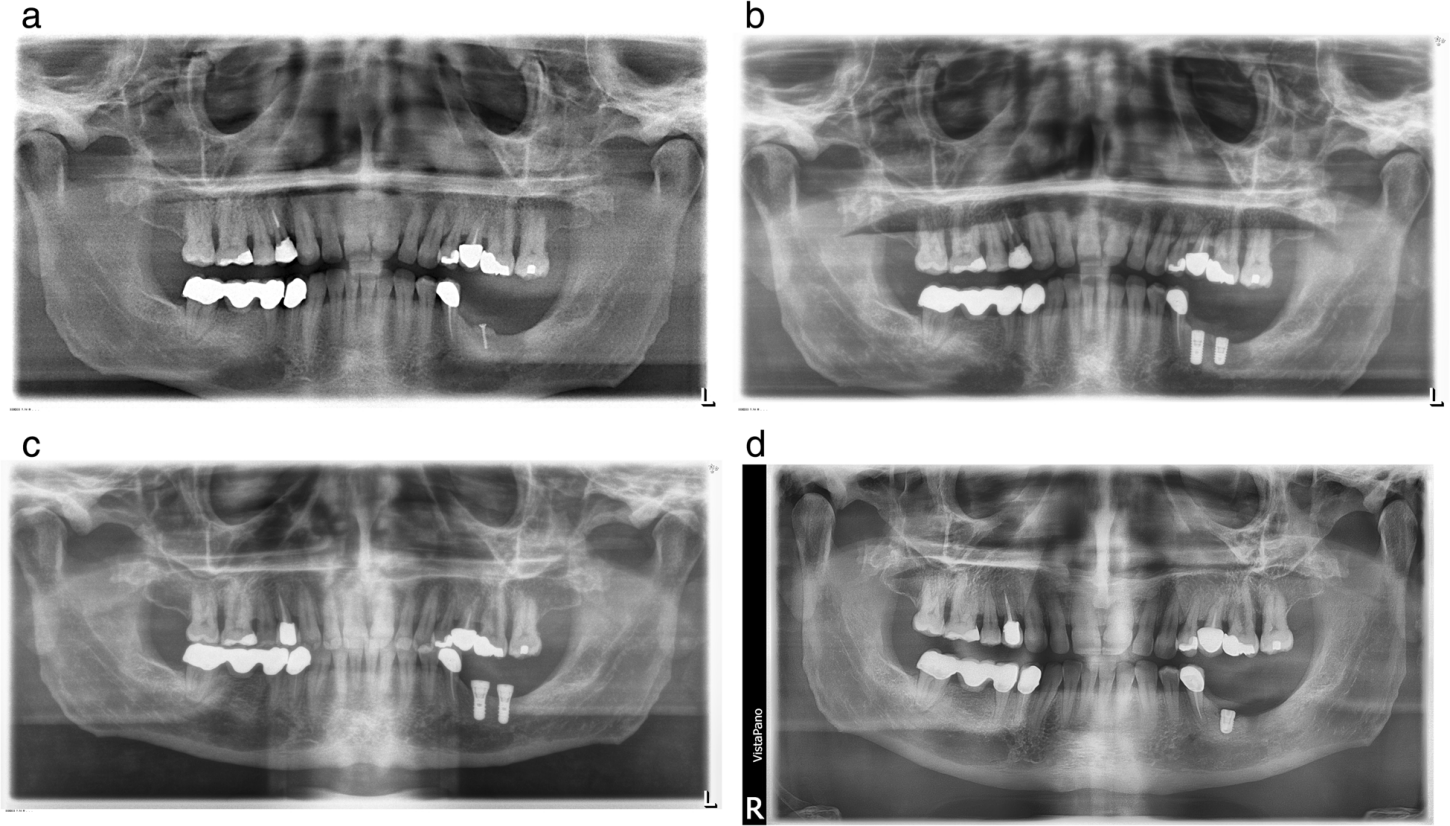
a Patient 1. Post grafting orthopantomogram. The bone block was secured with a single microscrew. b Patient 1. The radiograph demonstrates veritable inserted Straumann bone level implants after the first implant placement (1 day after implant placement). A peri-implant osteolysis is not visible. c Patient 1. Postoperative orthopantomogram (1 day after implant placement) after second implant placement of Straumann tissue level implants 6 months later. A peri-implant osteolysis is not visible. d Patient 1. Postoperative orthopantomogram after third implant placement (Conelog ScrewLine implant)
